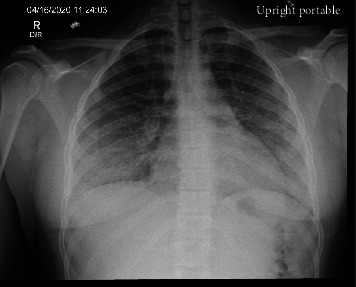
Chest X-ray: diffuse bilateral lung infiltrates with basilar predominance.
